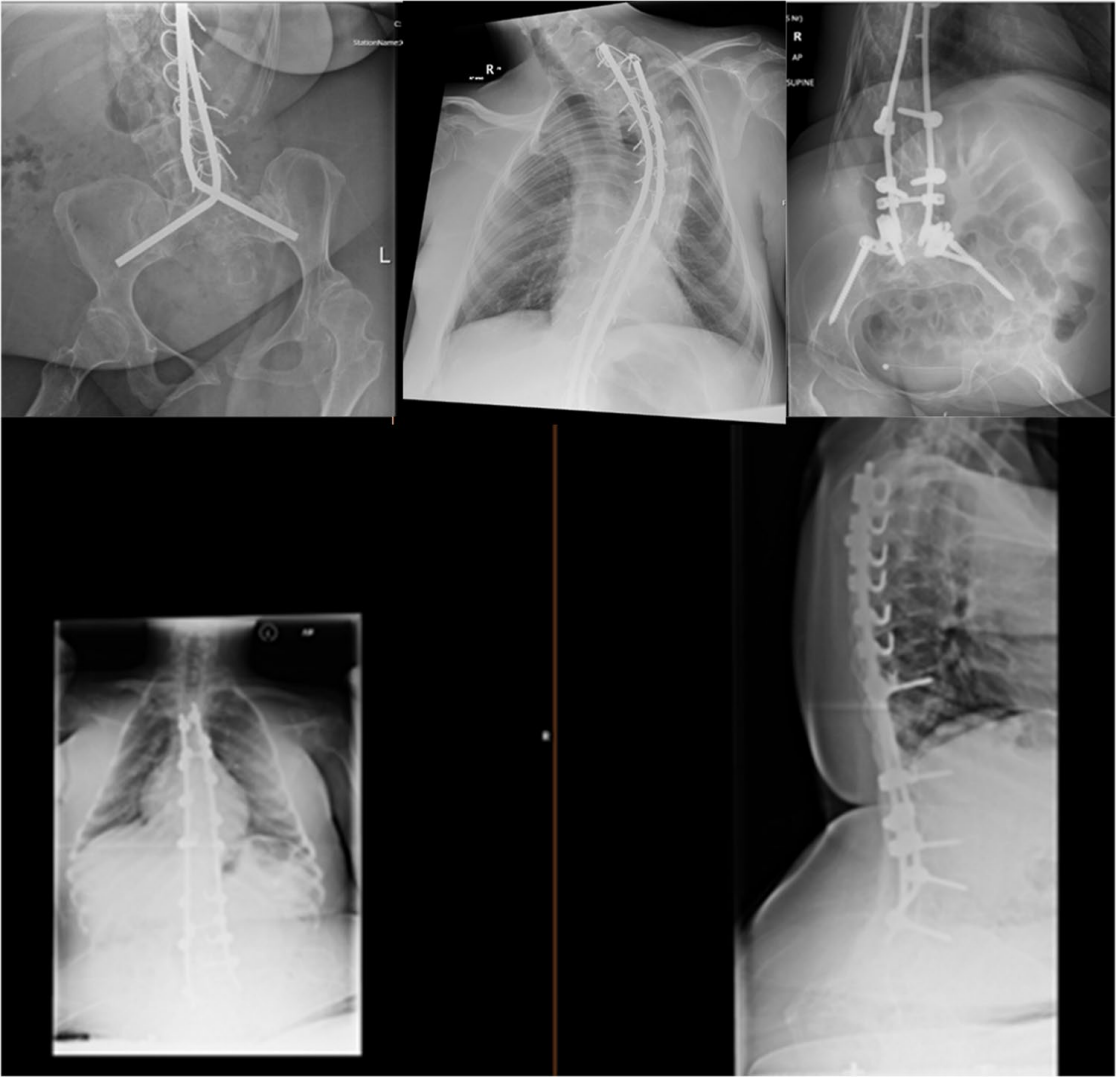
Lumbar spine x-ray images of five of the patients who had spinal fixation prior to pregnancy
